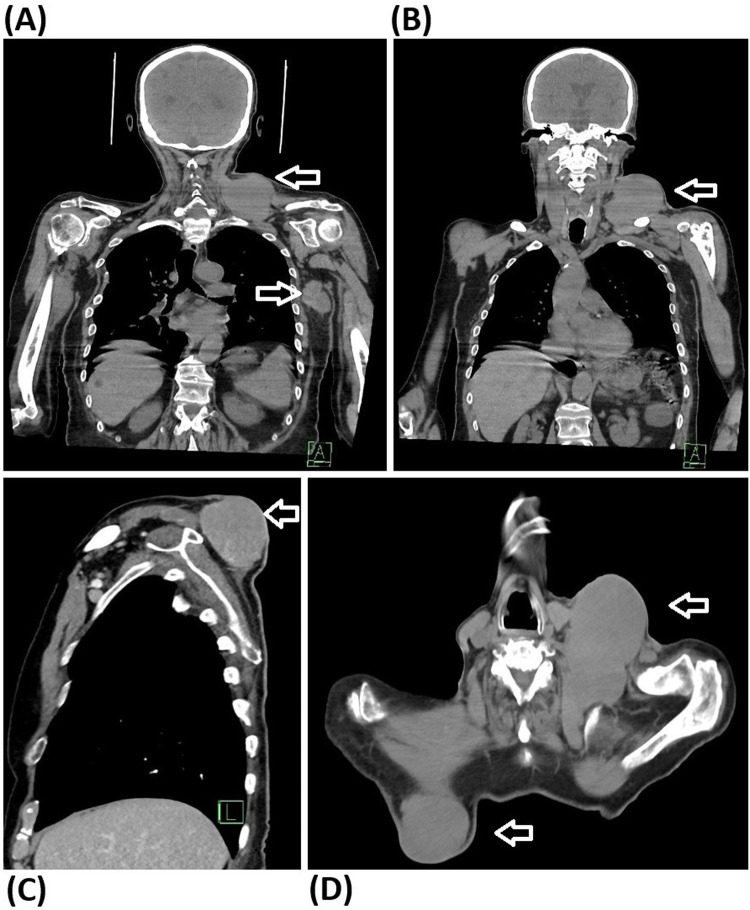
CT scan made 12 June 2025, imaging the location and extent of EMP lesions: (A) Coronal scan imaging the lesions above the left clavicle and in the left axilla. (B) Another coronal scan imaging dorsal parts of lesion above the left clavicle. (C) Sagittal scan imaging the well bordered lesion above the right scapula. (D) Axial scan imaging the lesion above the left clavicle in its extent invading to the deeper compartments of neck and chest, and the extent of lesion above the right scapula.
